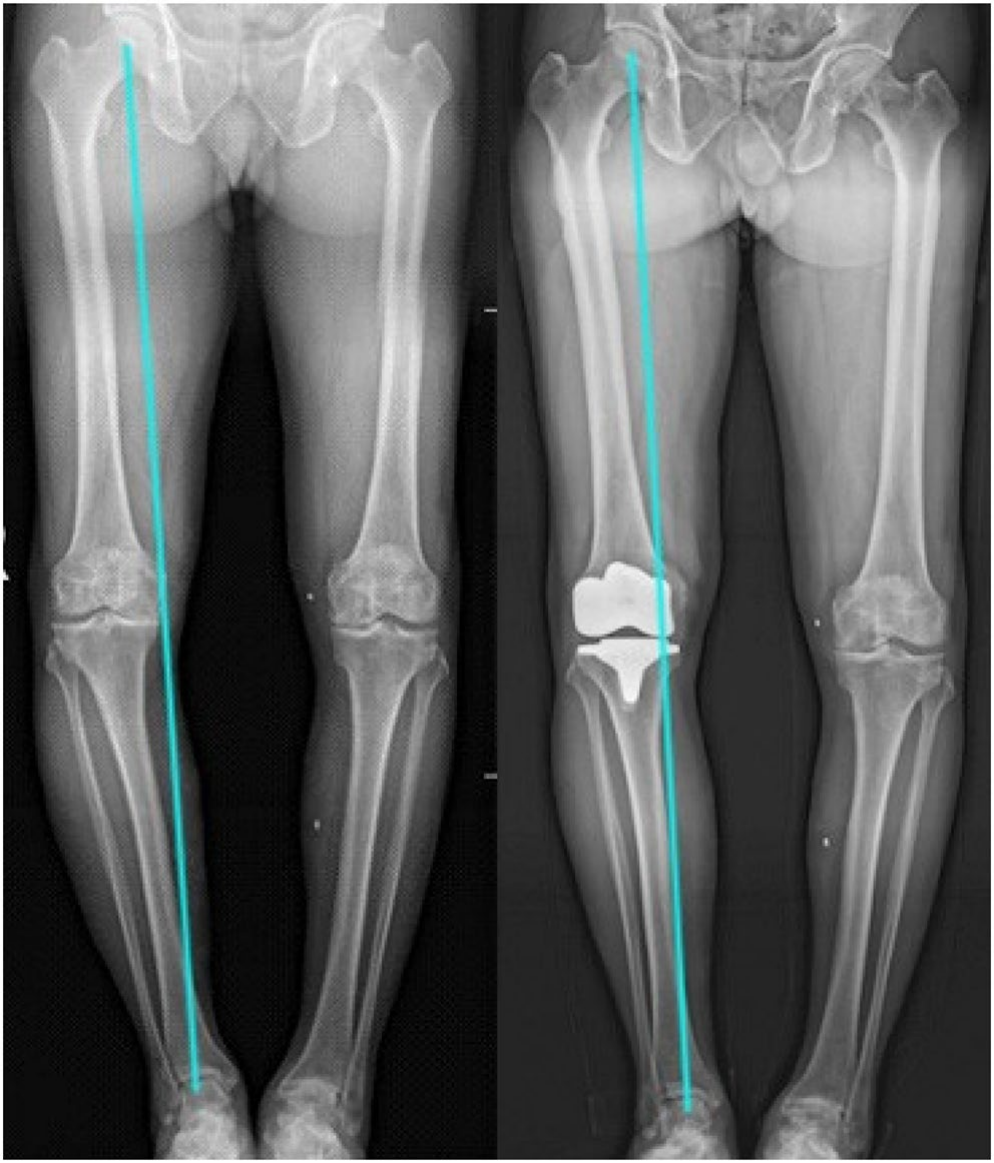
Postoperative long‐leg radiographs showing that KA‐TKA restored constitutional varus alignment. KA‐TKA, kinematic alignment‐total knee arthroplasty.
